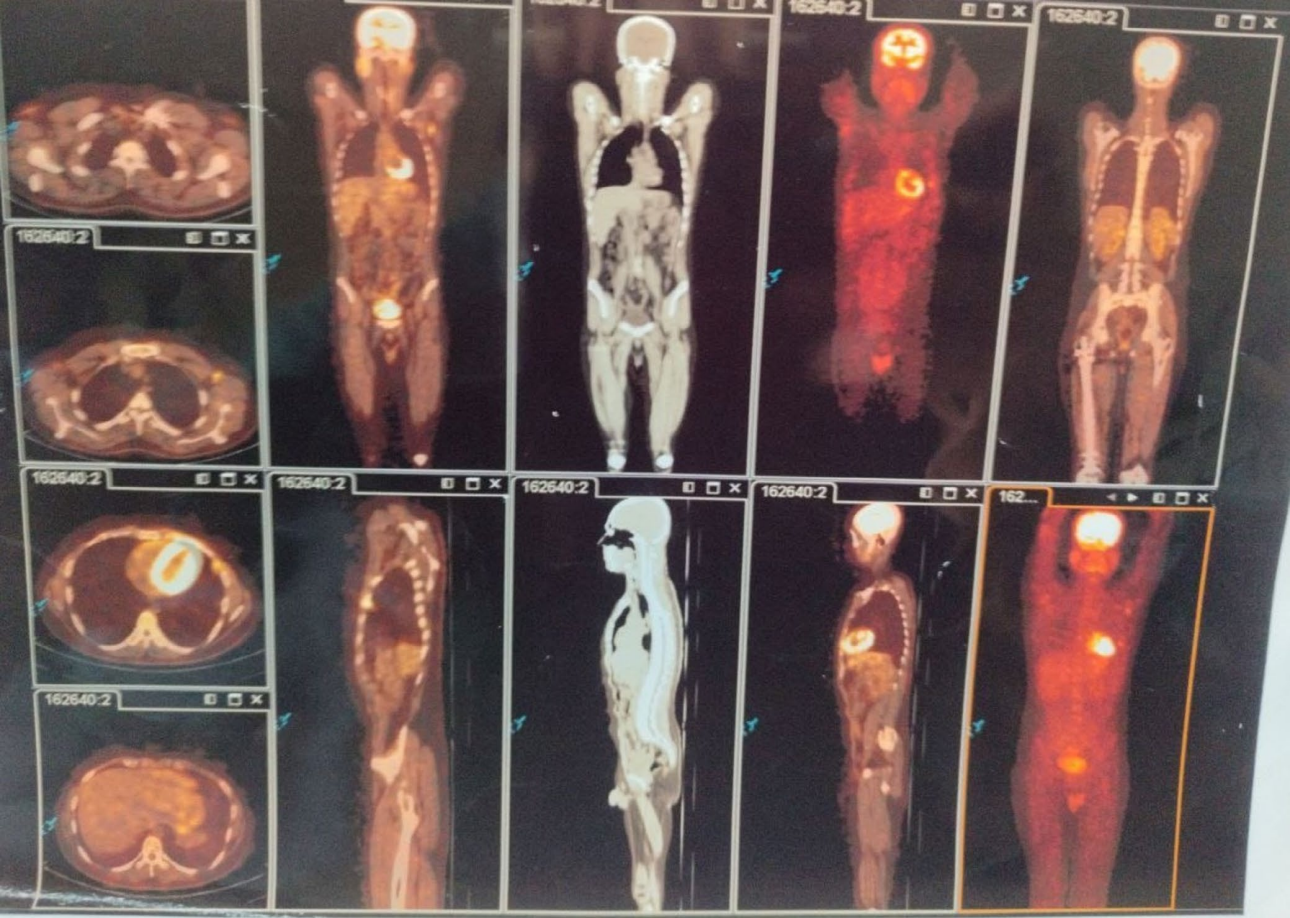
Whole‐body FDG PET/CT showing mild metabolic activity (SUV < 3) in the left subpleural pleural‐based lesion and small bilateral axillary lymph nodes, with no abnormal uptake in the cervical, mediastinal, abdominal, pelvic, or inguinal regions.
